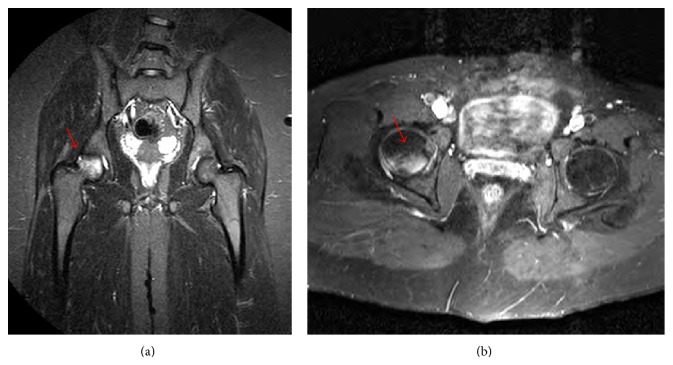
Hip MRI (a) coronal view and (b) axial view. A subcortical wedge shaped enhancing area (*arrows*) with adjacent bone marrow edema in the right femoral head posterior articular surface.
